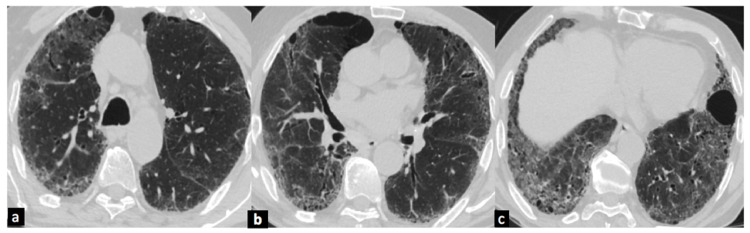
(a-c) HRCT chest showing marked improvement in ground-glass opacities in bilateral lung fields. HRCT, high-resolution computed tomography
